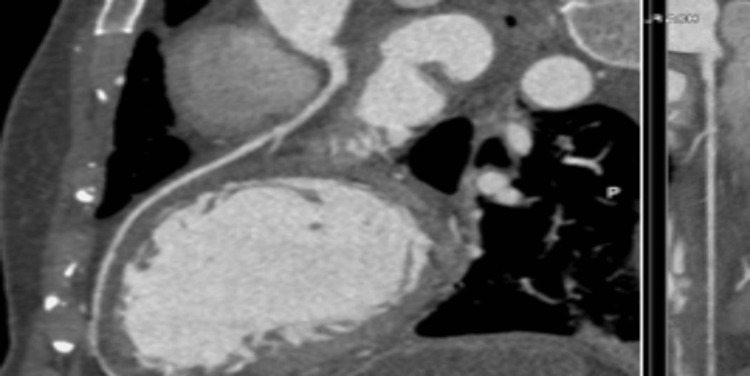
Left anterior descending artery. Coronary computed tomography angiography shows the left anterior descending artery being patent without any blockages or stenosis.
